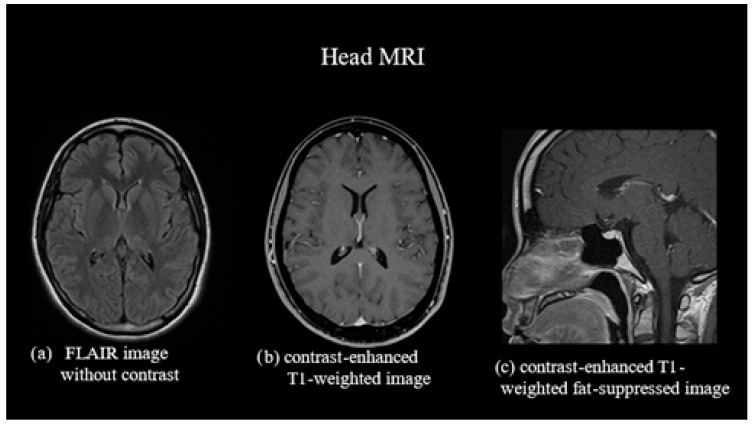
Magnetic resonance imaging (MRI) of the head. (a) Non-contrast fluid-attenuated inversion recovery image showing no subdural fluid accumulation. (b) Contrast-enhanced T1-weighted image showing no diffuse dural thickening. (c) Contrast-enhanced T1-weighted fat-suppressed image showing no dilation of the epidural venous plexus. FLAIR, fluid-attenuated inversion recovery.
